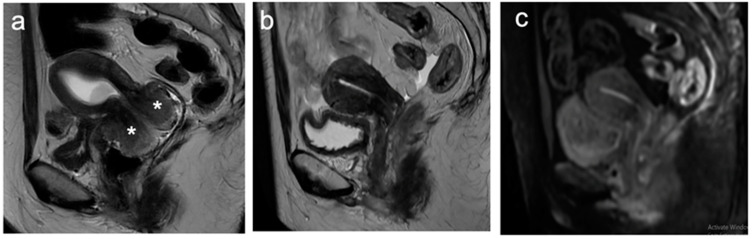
Baseline sagittal T2WI (a) shows an intermediate signal intensity bulky, exophytic cervical tumour (*). Sagittal T2WI (b) and DWI (c) after completion of chemoradiotherapy demonstrates significant reduction in tumour size with no residual abnormal signal intensity or diffusion restriction. This appearance indicates a complete response.
